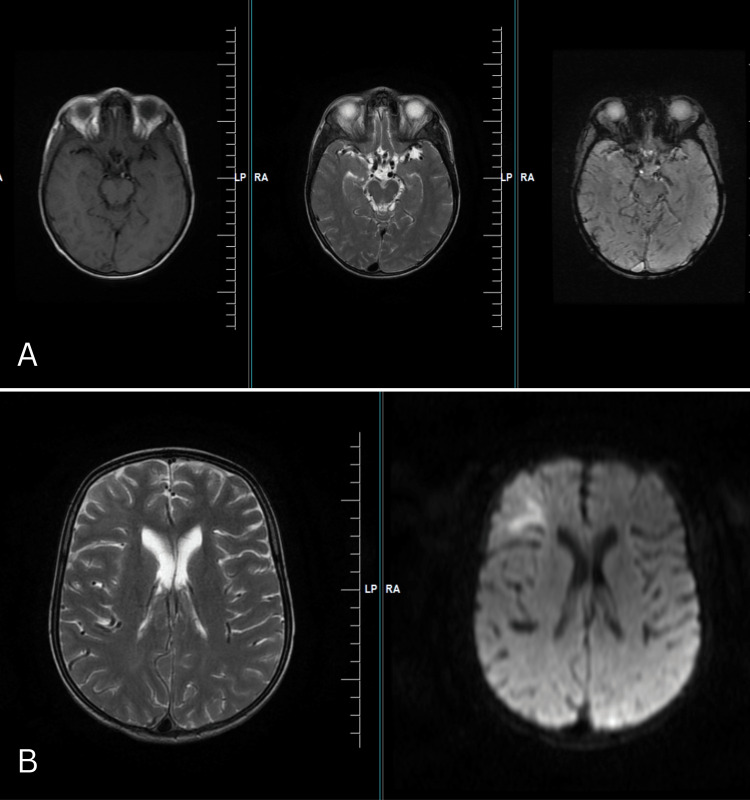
A) Focal subcortical hyperintensity in T2-weighted MRI in right frontal region showing restricted diffusion; B) Prominent dilated intracranial vessels with flow void on T1-weighted MRI and T2-weighted MRI, along with punctate blooming on GRE MRI - Magnetic resonance imaging GRE - Gradient recall echo
